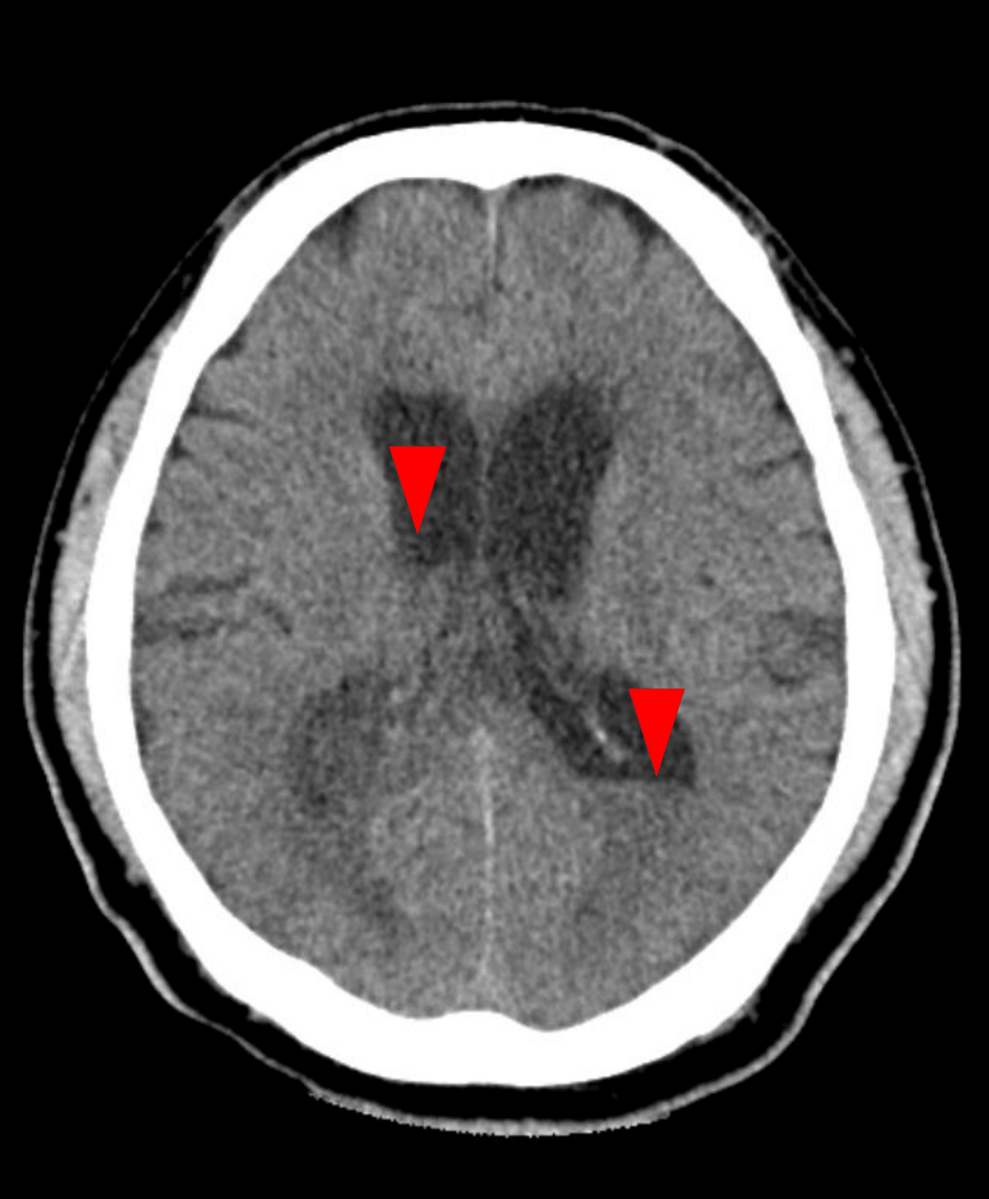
Brain CT on day three. A follow-up CT scan on day three revealed significant fluid accumulation in the bilateral lateral ventricles (red arrowheads).
